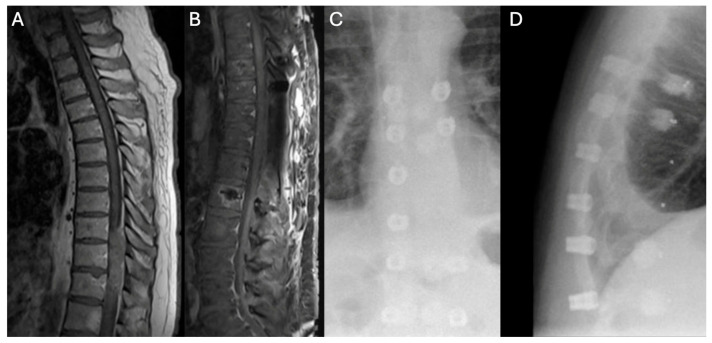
Case 2: pre- versus postoperative MRI. (A) Preoperative T1 post-contrast MRI showing enhanced T9–T12 extradural mass. (B) Postoperative T1 fat-sat post-contrast MRI showing removal of the enhanced tumor. (C) AP and (D) lateral chest X-rays showing the placement of the carbon-fiber pedicle screws and rods.
